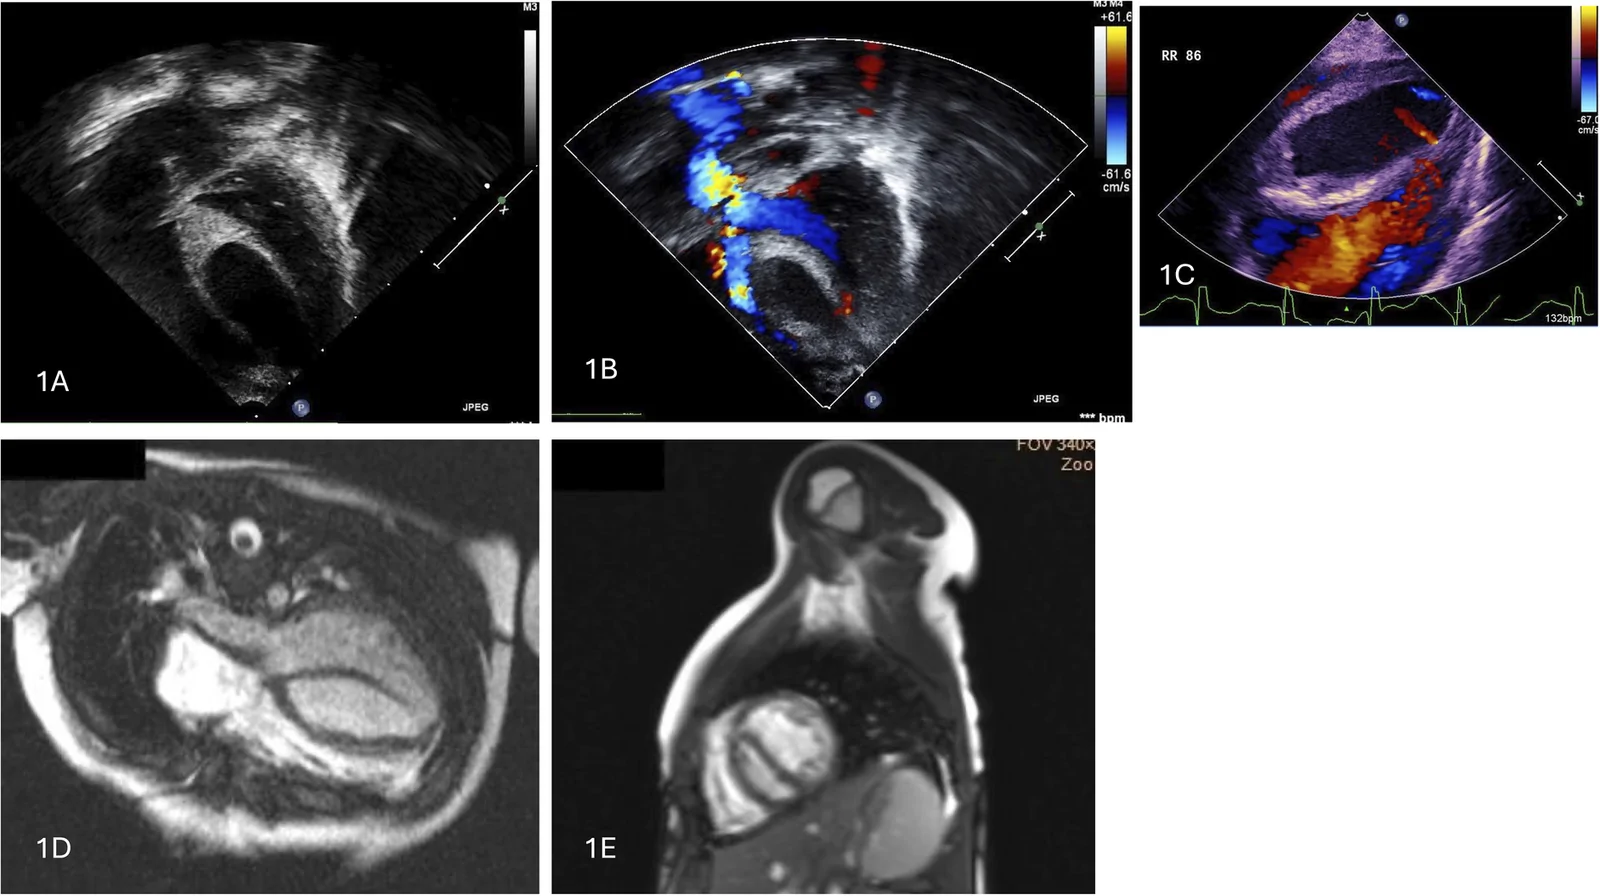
(A,B) Transthoracic echocardiography demonstrating a large intraseptal hematoma located within the interventricular septum, protruding toward both ventricular cavities. (C) Transthoracic echocardiography showing multiple small fistulous communications between the interventricular septal hematoma cavity and the left ventricular lumen. (D,E) Cardiac magnetic resonance imaging demonstrating dissected interventricular septal myocardium with communication to the left ventricular cavity at the apical septal segment.
